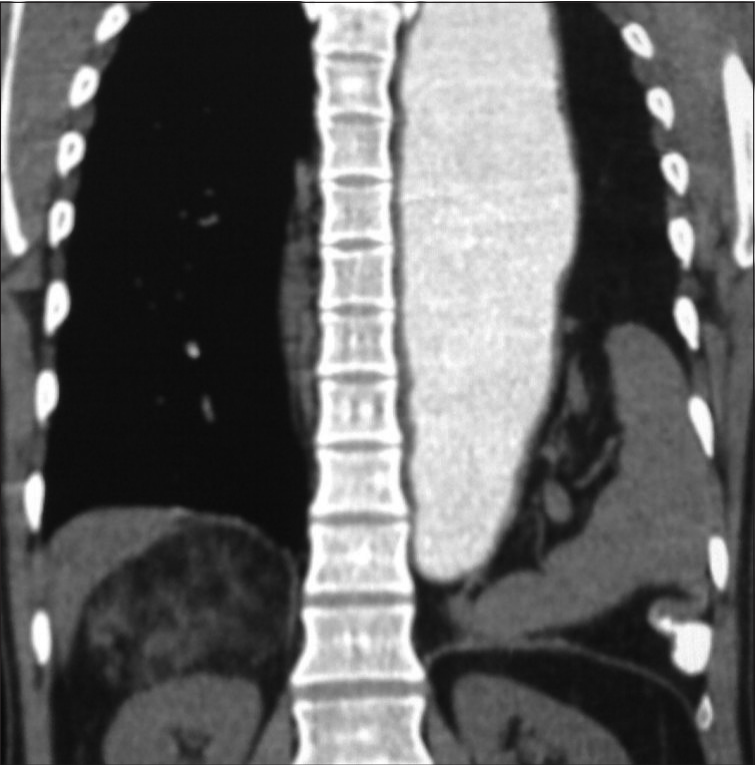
Coronal reformation CT shows the whole of stomach with luminal contrast, multiple small intestine coils, colon and spleen above left hemidiaphragm inside left hemithorax; fat attenuation mass with more dense areas inside is seen in the right adrenal gland
